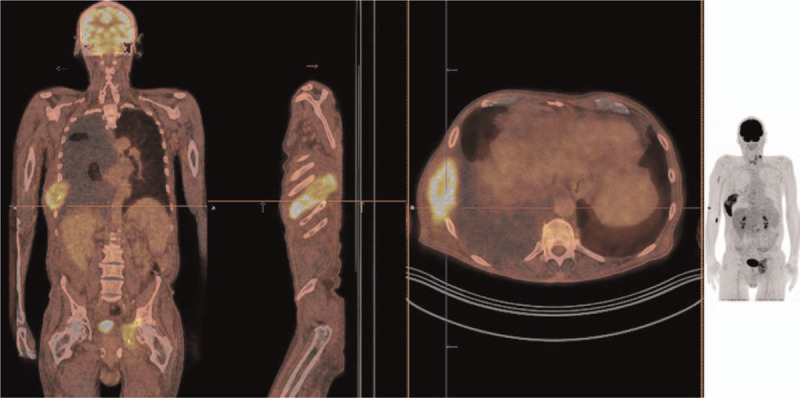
18F-PET/CT After thyroidectomy and before the 8th rib resection (February 2018).
